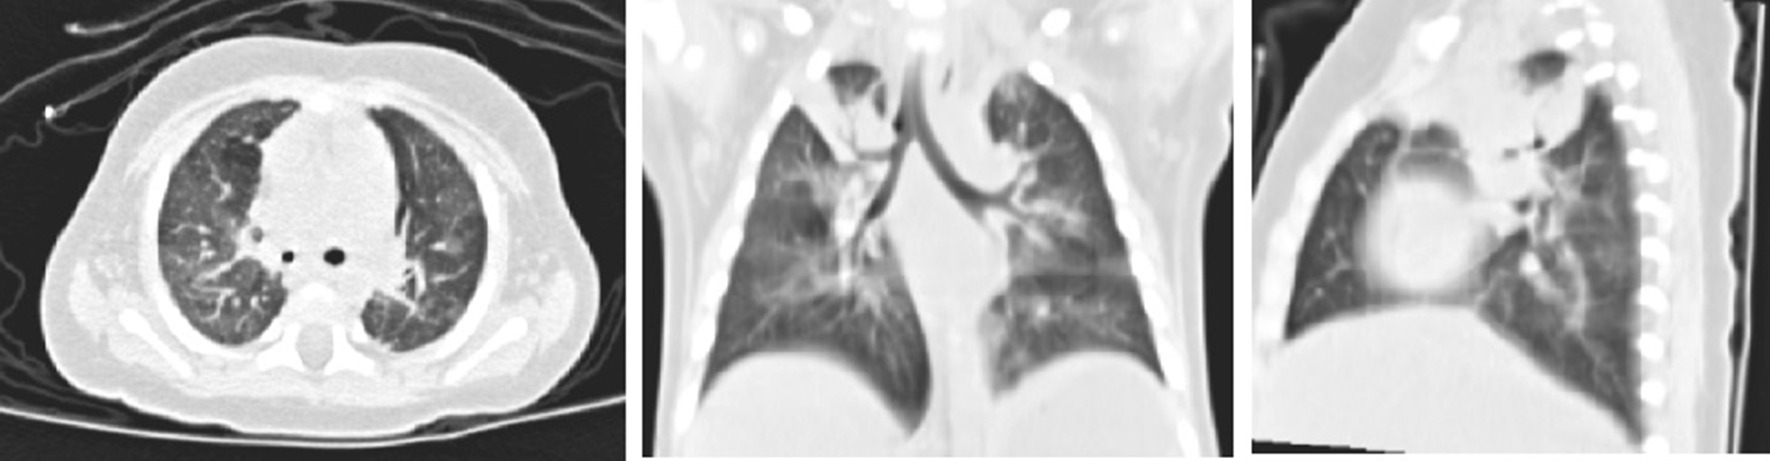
High-resolution CT scan of the chest revealing diffuse infiltration and areas of consolidation in the right upper in a 4-month-old girl
